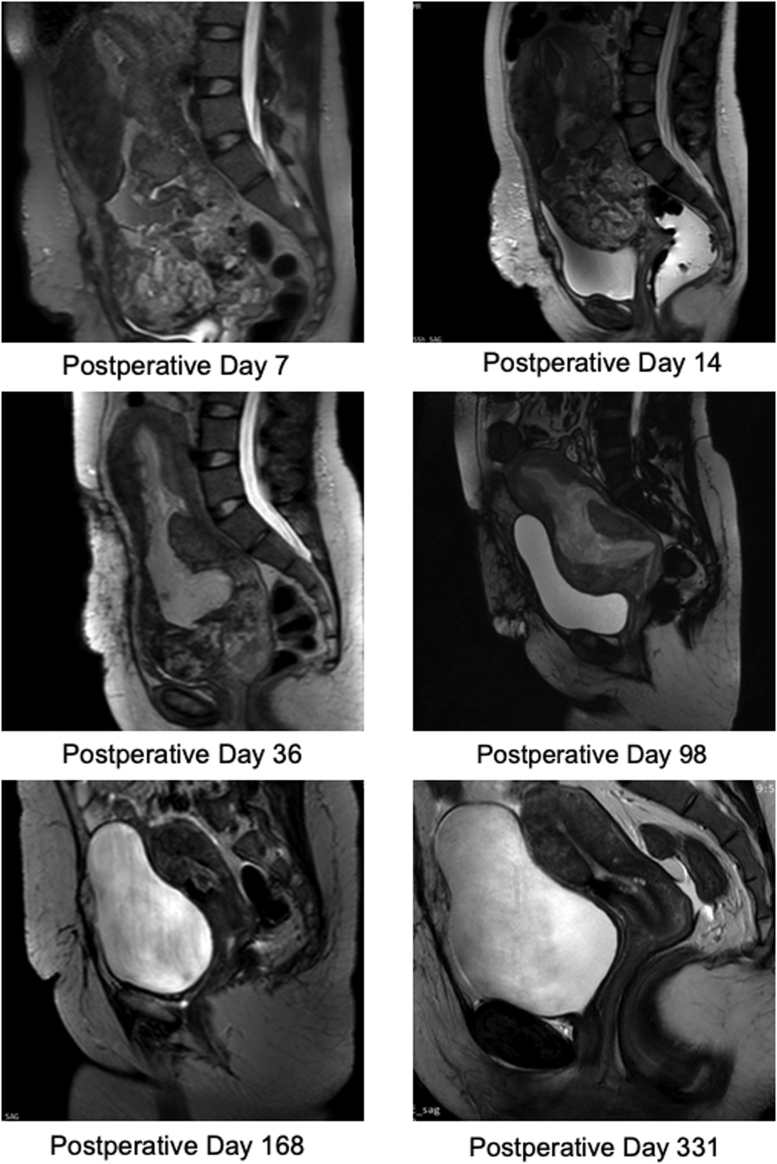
Serial magnetic resonance imaging (MRI) of conservative management of placenta percreta.
